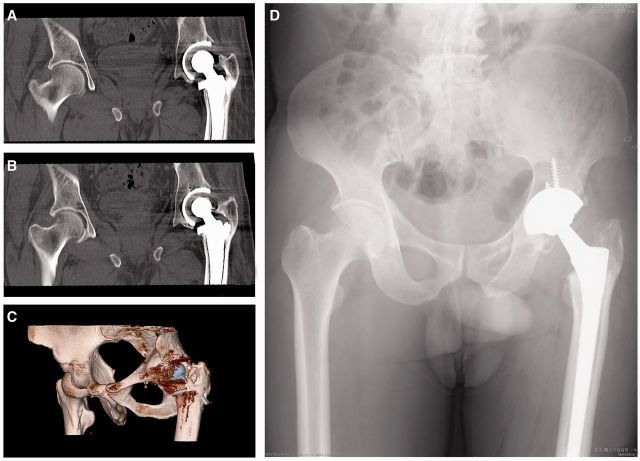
A 65-year-old man developed femoral components loosening 6 years after the operation. Hip CT scan and three-dimensional reconstruction graphs showed bone defects of proximal femur (A–C). MC grafts were used to reconstruct the bone defects and radiographs taken at 3rd month (lost) and 12th month (D) showed that the proximal femur bone grafts were well remodeled
